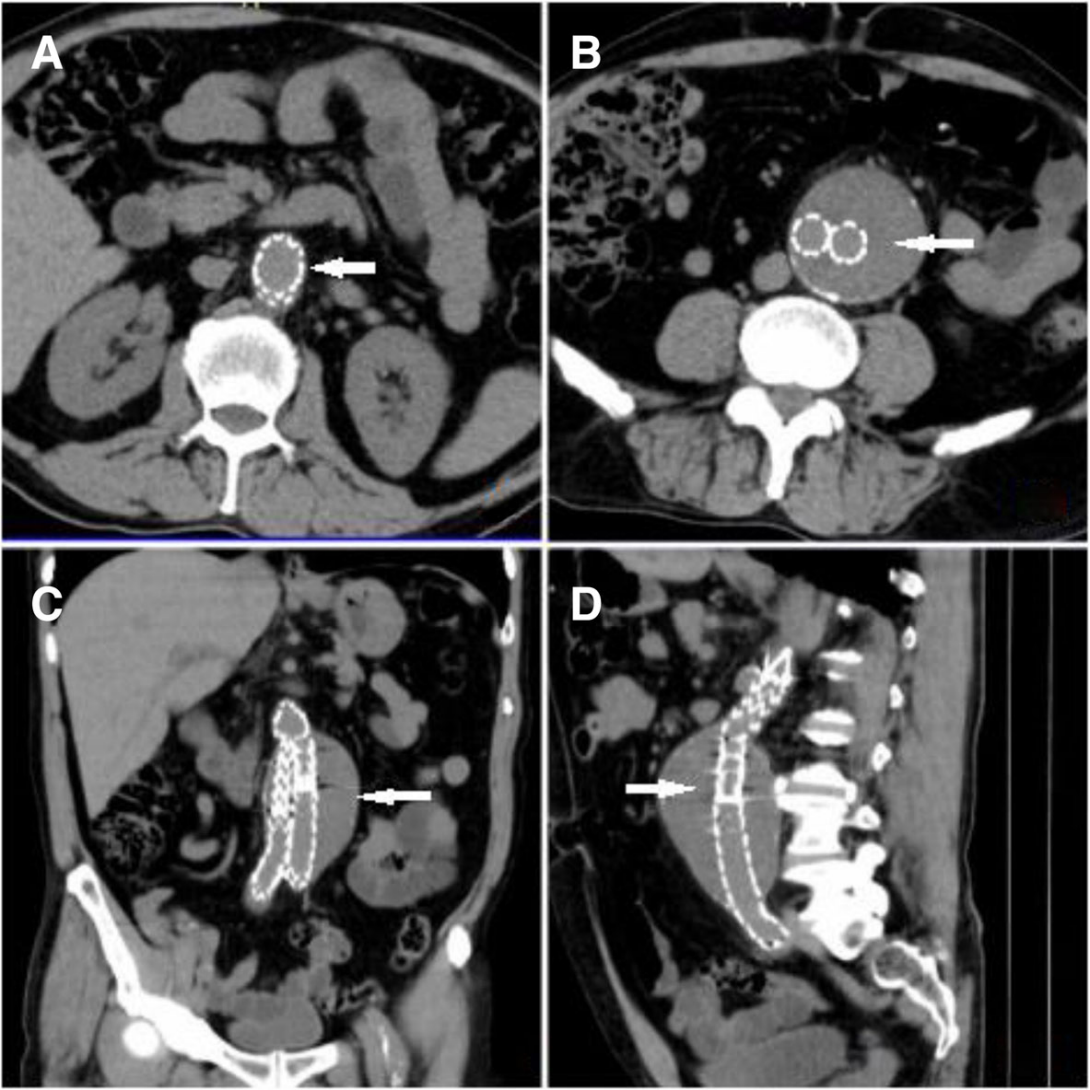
a Abdominal computed tomography transverse section after endovascular surgery. Endovascular prosthesis implantation is observed. b Aneurysmal stent implantation is observed. c Coronal section with implantation of prosthesis. d Sagittal section with implant of prosthesis. The prosthesis is marked with *white arrows*
